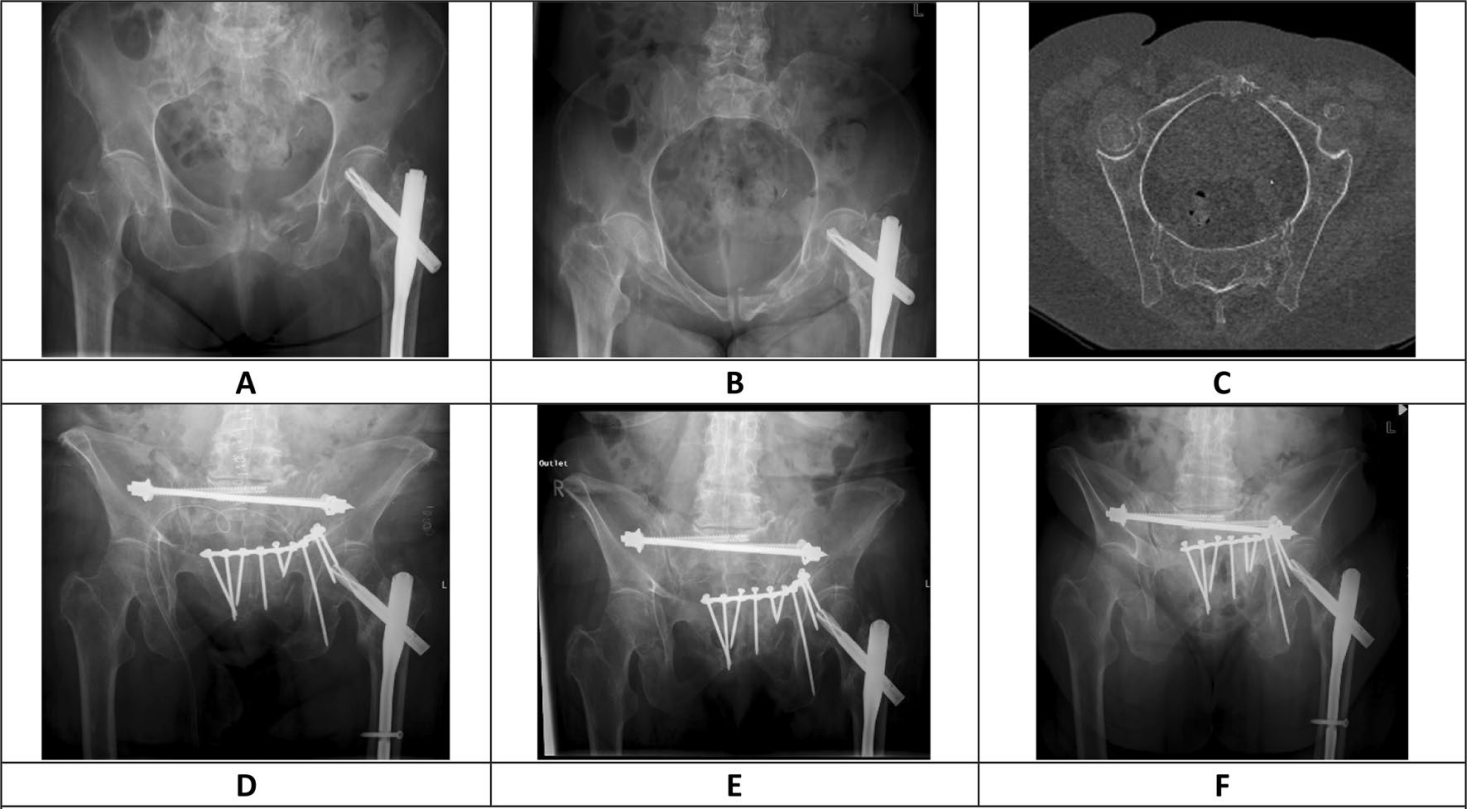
Shows a 85 year old patient with a chronic FFP 4b fracture with a largely displaced fracture of left anterior pelvic ring in the Nakatani II region. (a Conventional AP radiograph, b conventional inlet radiograph c CT reconstruction pelvic brim view). She was treated with a sacral bar and bilateral SI screws posteriorly and a ten-hole SPO with unilateral infra- and supra-acetabular screws. The first screw loosening was observed within the first week after treatment (d 1 week post-operative conventional outlet radiograph) and progressive loosening of supplementary screws over the following 4 months. (e 4 weeks post-operative conventional outlet radiograph, f 4 months post-operative conventional outlet radiograph). The fracture healed and the patient did not require any revision surgery
